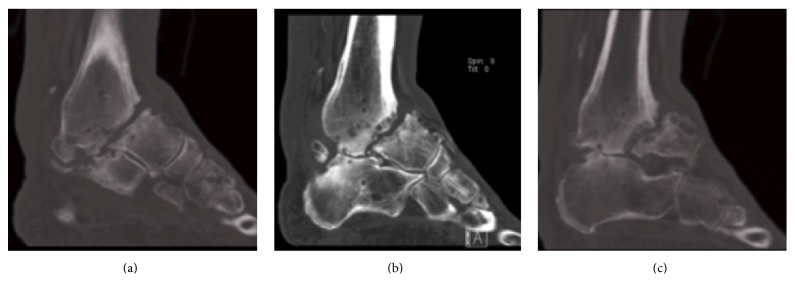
CT scan at presentation: sagittal slices demonstrate size of distal fragment. (a) Medial slice, (b) central slice, and (c) lateral slice.
